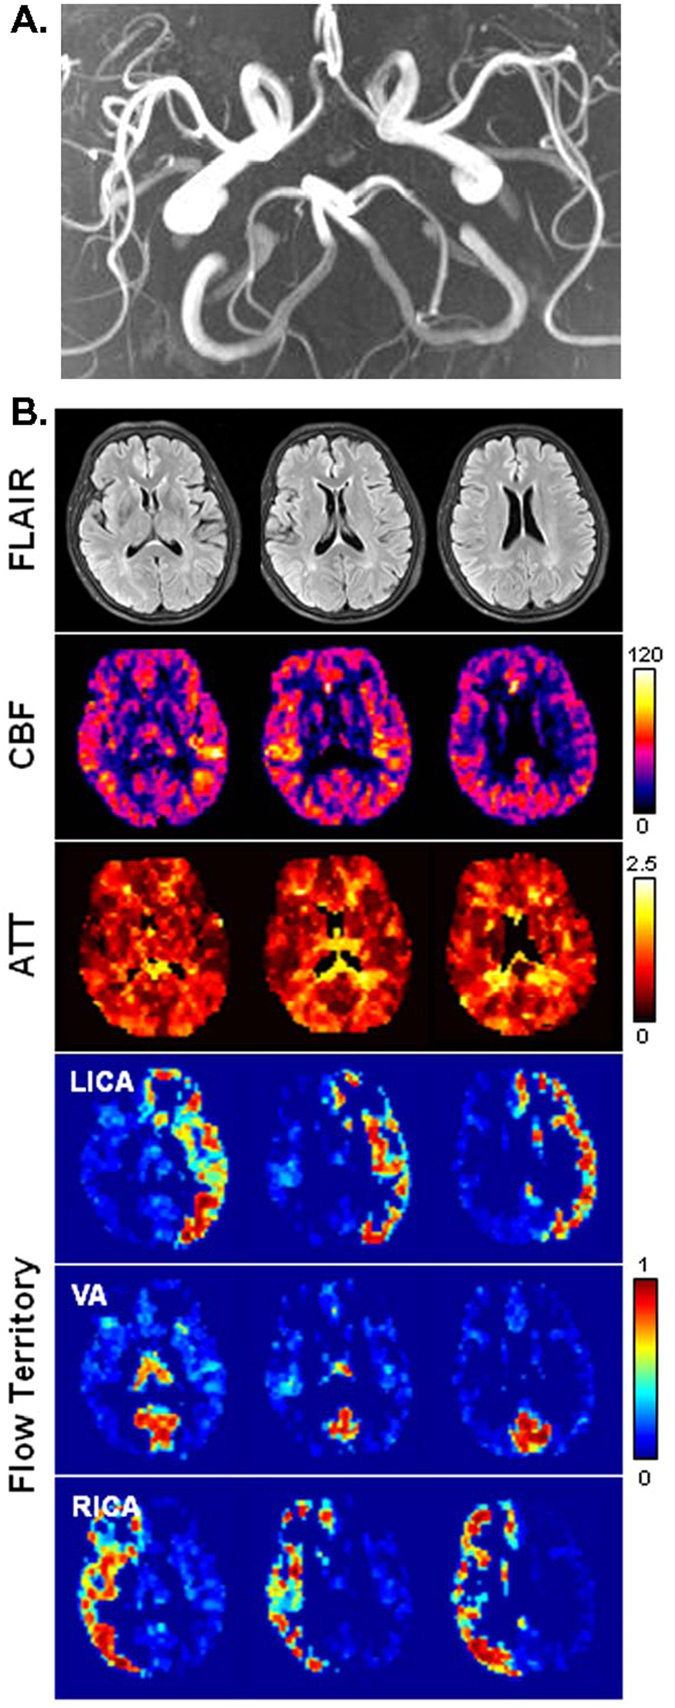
MR images obtained from a 65-year-old female patient with asymptomatic ICA steno-occlusive disease (10–30% stenosis in the left ICA and no stenosis in the right ICA). (A) Time-of-flight angiography. Projection view from below. (B) From top to bottom rows (3 slices are shown in radiological convention): FLAIR images, CBF maps (in units of ml-blood/100 ml-tissue/min), ATT maps (in units of sec), and flow territory maps. Flow territory maps show the membership levels generated by fuzzy clustering, which range from 0 to 1.
